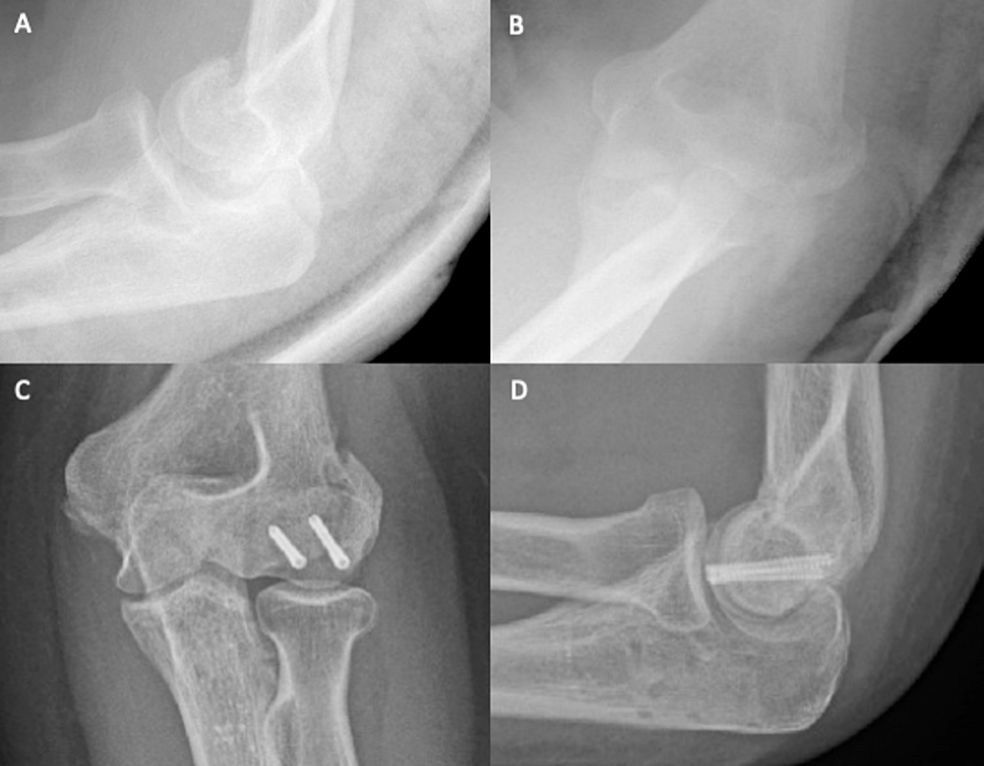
Plain elbow X-rays of case 5. Figure 7A, 7B shows anteroposterior and lateral views, respectively, of a type I capitellum fracture. The backslab applied in the emergency department can be seen. Figure 7C, 7D shows anteroposterior and lateral views, respectively, of the elbow after ORIF. ORIF: open reduction and internal fixation
